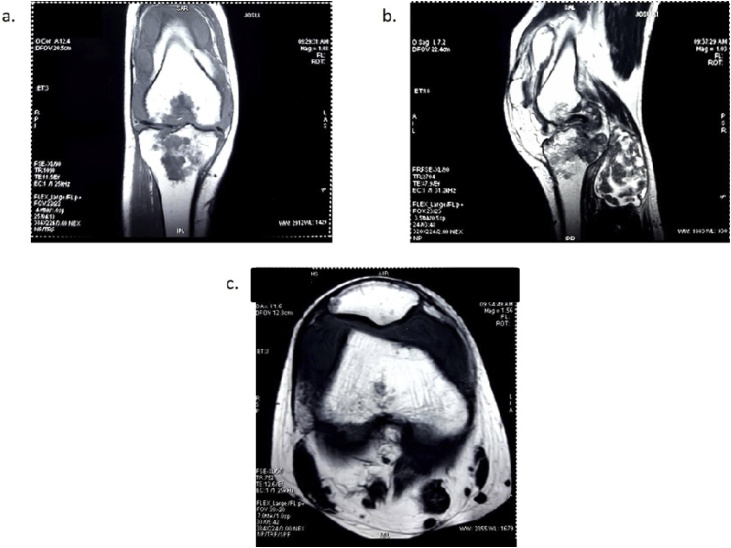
MRI examination of the knee. (a) Based on coronal view, it showed well-defined iso-hyperintensed lesion and fat-set epimetaphysis femur (distal); tibia-fibula epimetaphysis (proximal); half of right inferior patella pole. (b) In sagittal T2 posterior projection of right genu, there was an iso-hyperintense mass that involved half of musculus gastrocnemius (mediolateral), plantaris, popliteal, and half of femoral arterial and venous and tibial nervesagittal view. (c) From the axial view, there was a free fluid collection in which dominant in suprapatella and minimal infrapatella projection.
